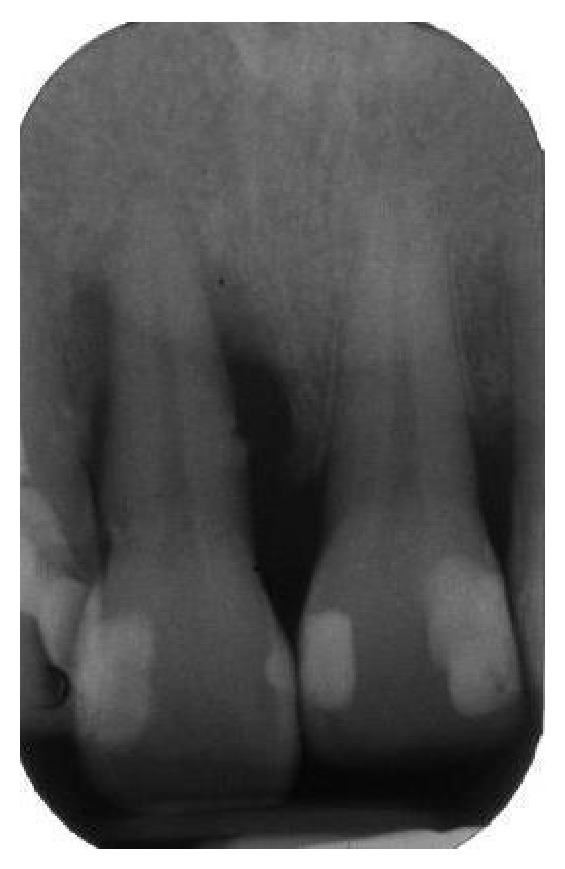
The periapical radiograph revealed a localized, severe bone resorption affecting the tooth.
